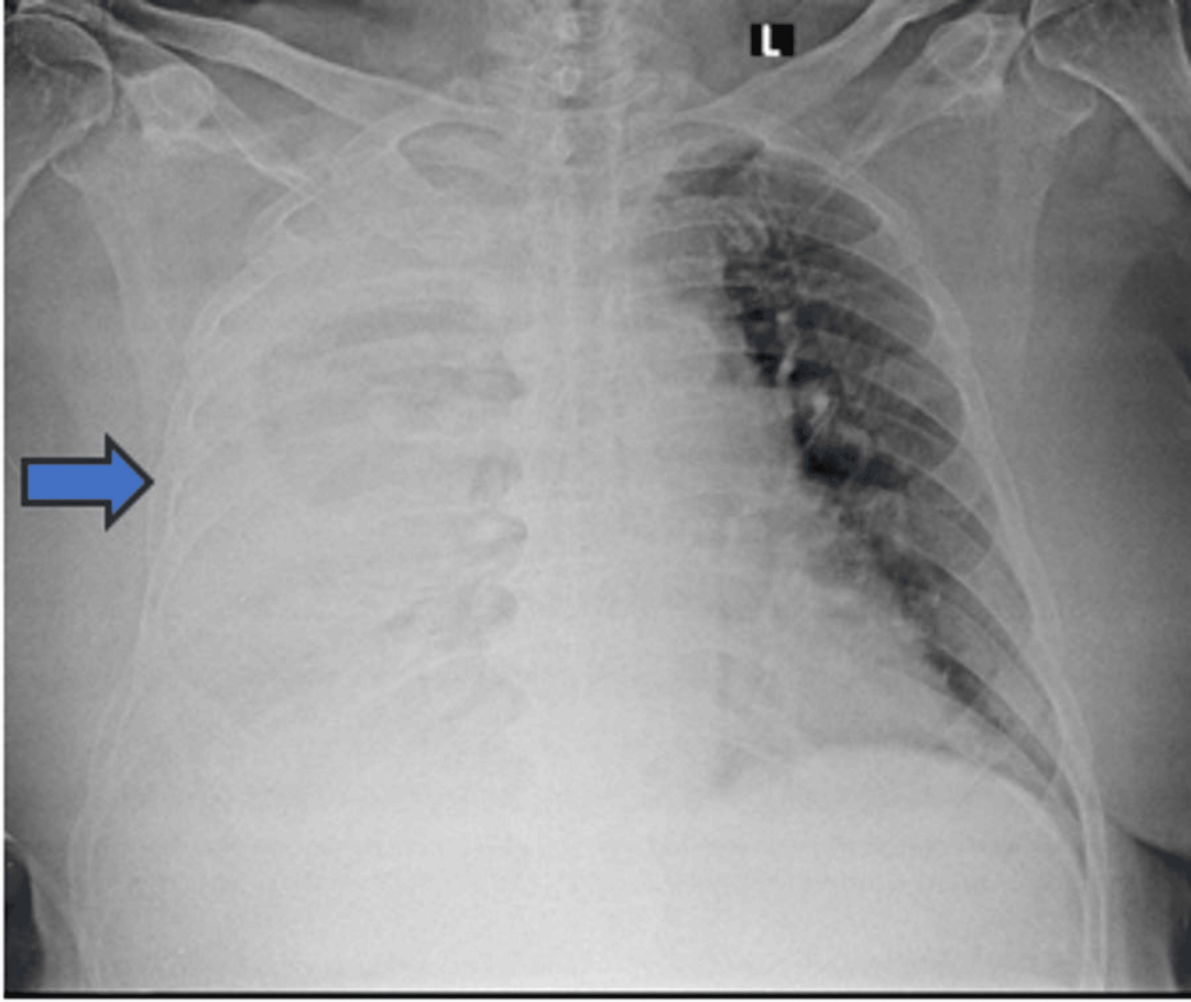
Radiograph of chest showing moderate pleural effusion (blue arrow).
